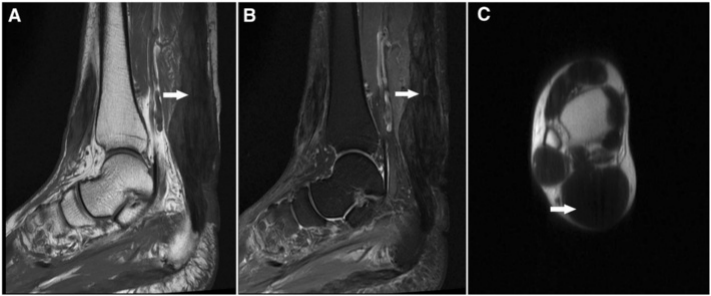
5 MRI results: tendon. MRI of both ankles. Sagittal long-T1-weighted image (A, arrow) and short-T2-weighted image (B, arrow), and axial long-T1-weighted image (C, arrow) of fusiform thickenings in the Achilles tendons in a patient with CTX.
